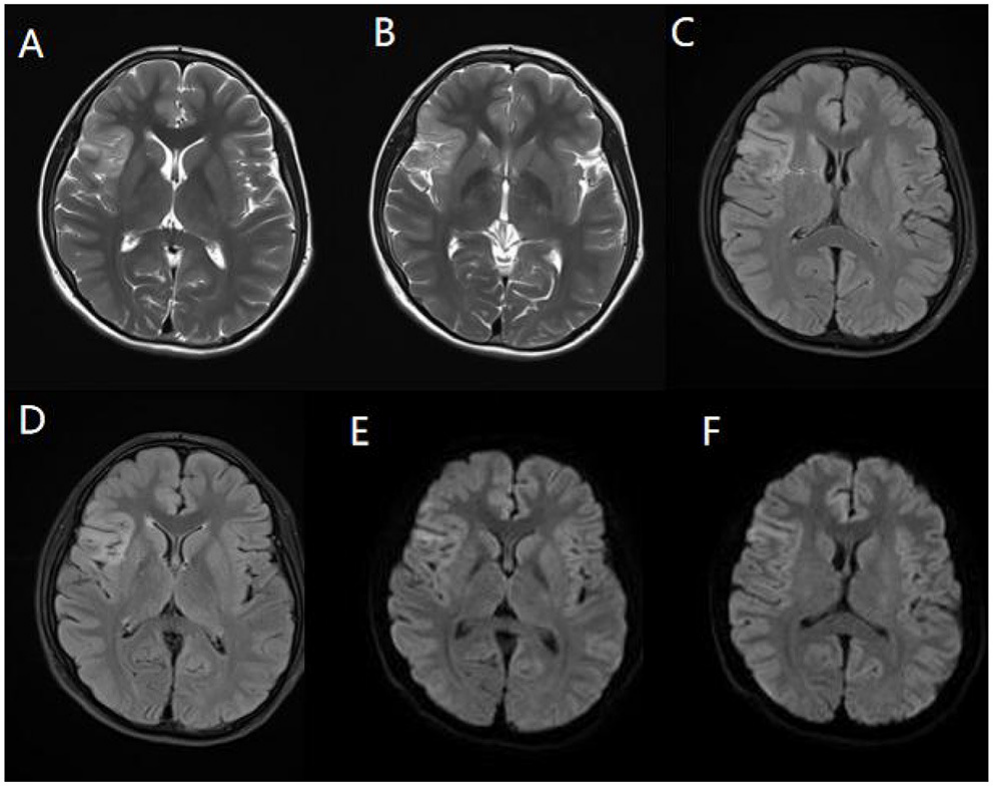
Magnetic resonance imaging (MRI) of a representative pediatric patient admitted for convulsions for 2 weeks and diagnosed with anti-NMDA receptor encephalitis (NMDA receptor antibody in CSF was 1:100). Right temporal and insula lobes and frontal cingulate gyrus showed lamellar abnormal signasl. T2WI showed a high signal (A,B). FLAIR sequence was dominated by a high signal (C,D), and DWI showed a high signal (E,F).
